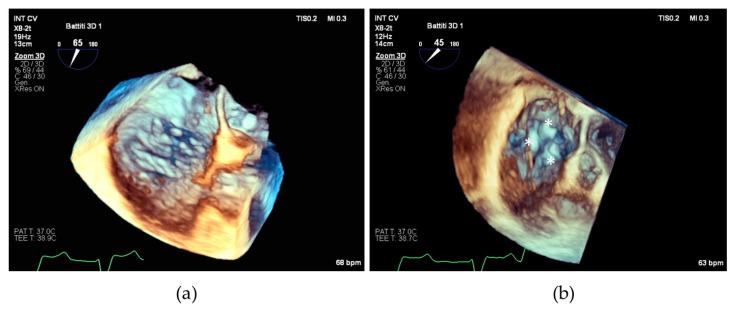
(a) Transesophageal echocardiographic in the 3D zoom modality showing complex tricuspid valve morphology; (b) the same valve after repair with implantation of three devices individuated by asterisks.
